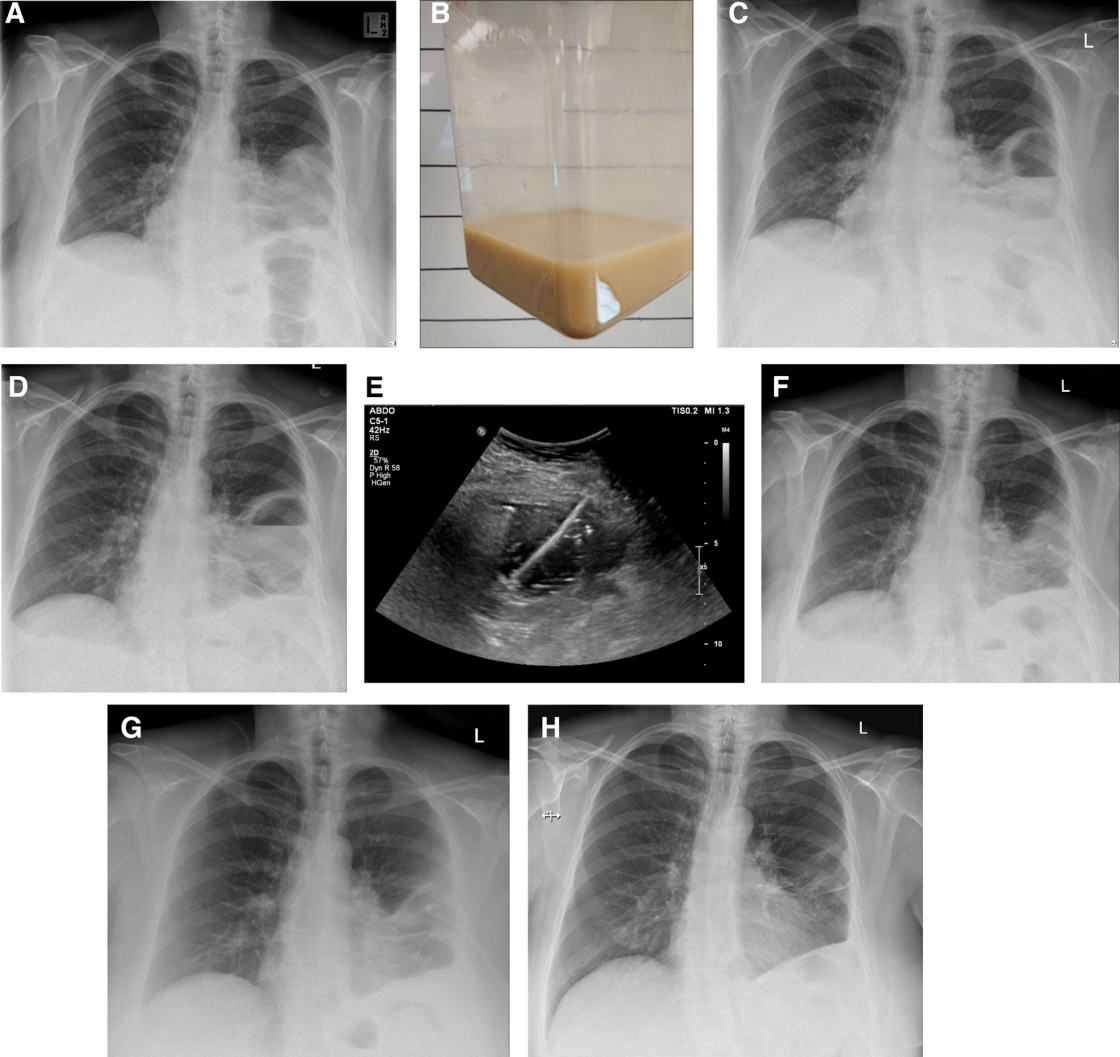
(A) The basal collection cleared with tissue plasminogen activator/deoxyribonuclease (tPA/DNase) therapy but the lateral locule enlarged with time and (B) frank pus was aspirated via a 5‐F catheter. However, the fluid reaccumulated over the following few days (C). A 12‐F chest drain was inserted under real‐time imaging guidance and Seldinger technique (D). The position of the drain was confirmed with ultrasound (US) (E) and chest X‐ray (CXR) (F). The chest radiograph showed near‐complete clearance of the lateral locule after the incidence of migration of the intrapleural tPA dose to the lung and subsequent significant coughing (G). Follow‐up radiograph after three weeks demonstrated continued resolution of the collections (H).
